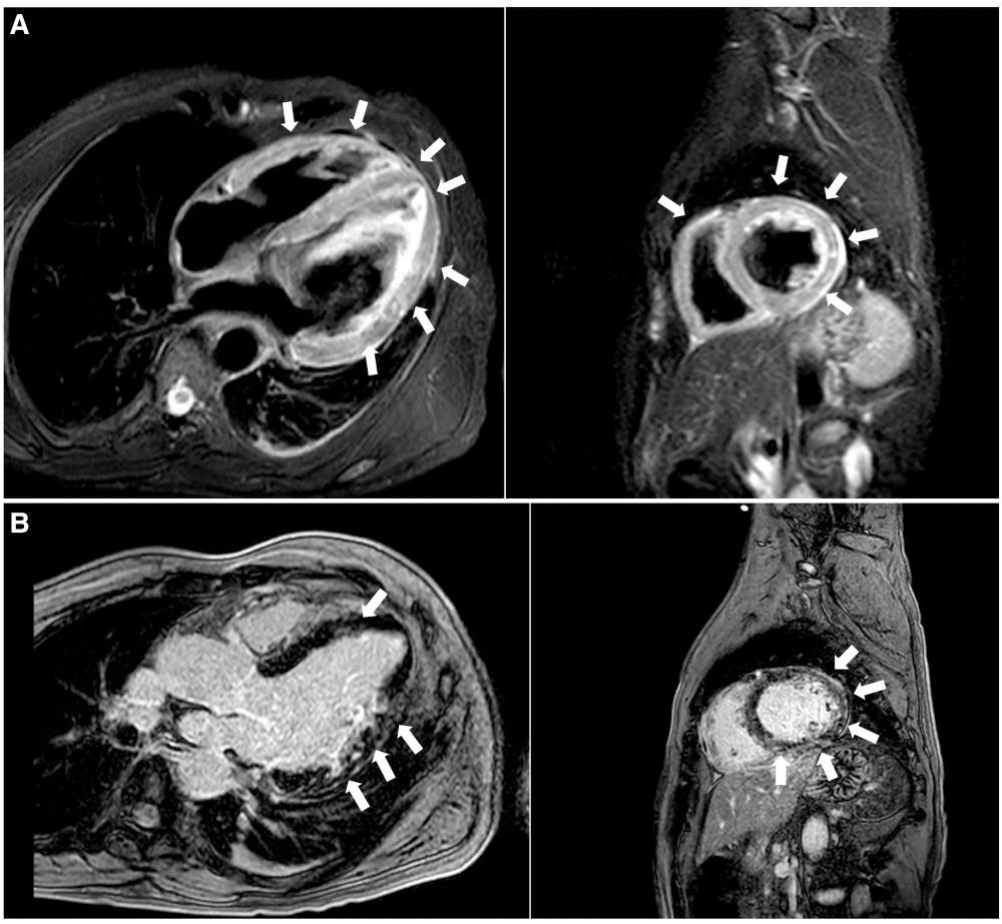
Cardiac magnetic resonance imaging. (*A*) Long- and short-axis T2-weighted short tau inversion recovery images showed diffuse myocardial hyperintensity (marked with arrows) consistent with widespread myocardial oedema, with more intense patchy areas in the basal-to-mid-lateral wall (mean T2 relaxation times, 69 ms; normal ≤ 50 ms) (*Figure 2A*). Additional tissue characterization parameters were consistent with widespread myocardial tissue injury (mean T1 relaxation times, 1172 ms; normal < 1045 ms; mean elevated extracellular volume fraction, 44%; normal < 27%). (*B*) In the long- and short-axis late gadolinium enhancement sequences, multiple striae and patchy areas of late gadolinium enhancement with non-ischaemic distribution (mid-wall, subepicardial, and septal) were identified, particularly in the basal-to-mid-lateral wall. Late gadolinium enhancement, highlighted by arrows, is evident in the basal to mid-lateral and inferolateral segments of the left ventricular myocardium (*Figure 2B*).
